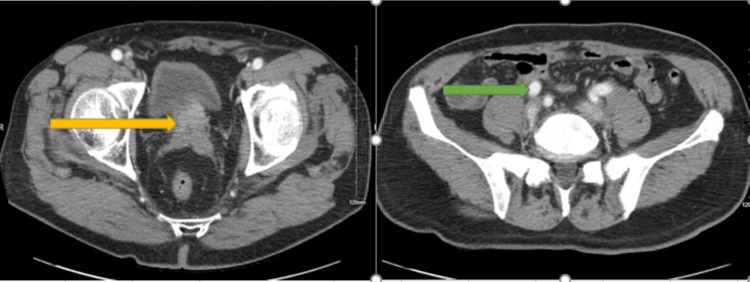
CT abdomen and pelvis with contrast showing an enlarged prostate (yellow arrow) and retroperitoneal lymphadenopathy (green arrow)
